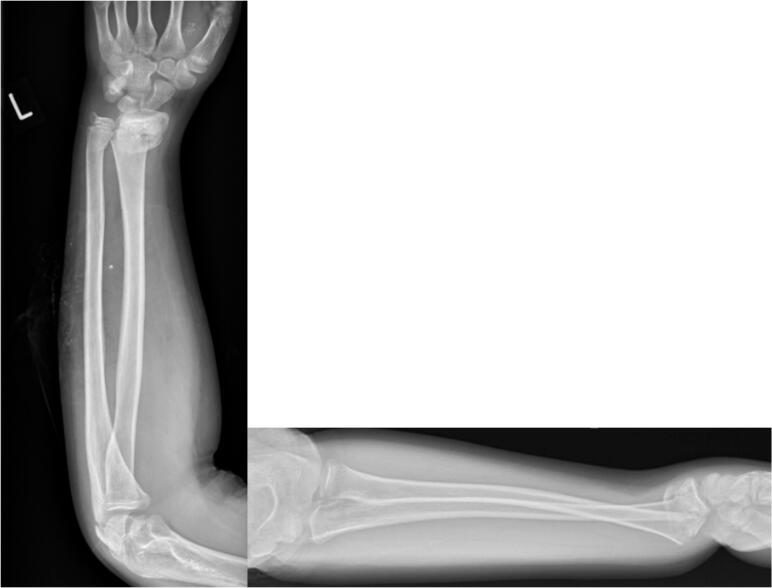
AP and lateral radiograph at initial presentation showing distal third radius & ulna fracture.
